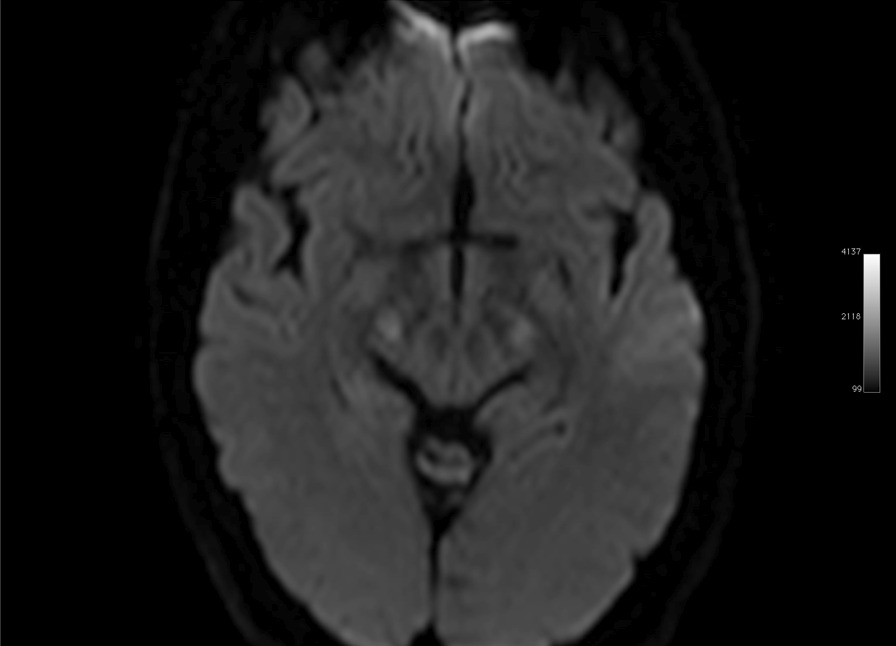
Axial DWI demonstrates T2 shine-through characteristics with high signal in the corticospinal tracts noted on the DWI with isotense signal on the apparent diffusion co-efficient map
